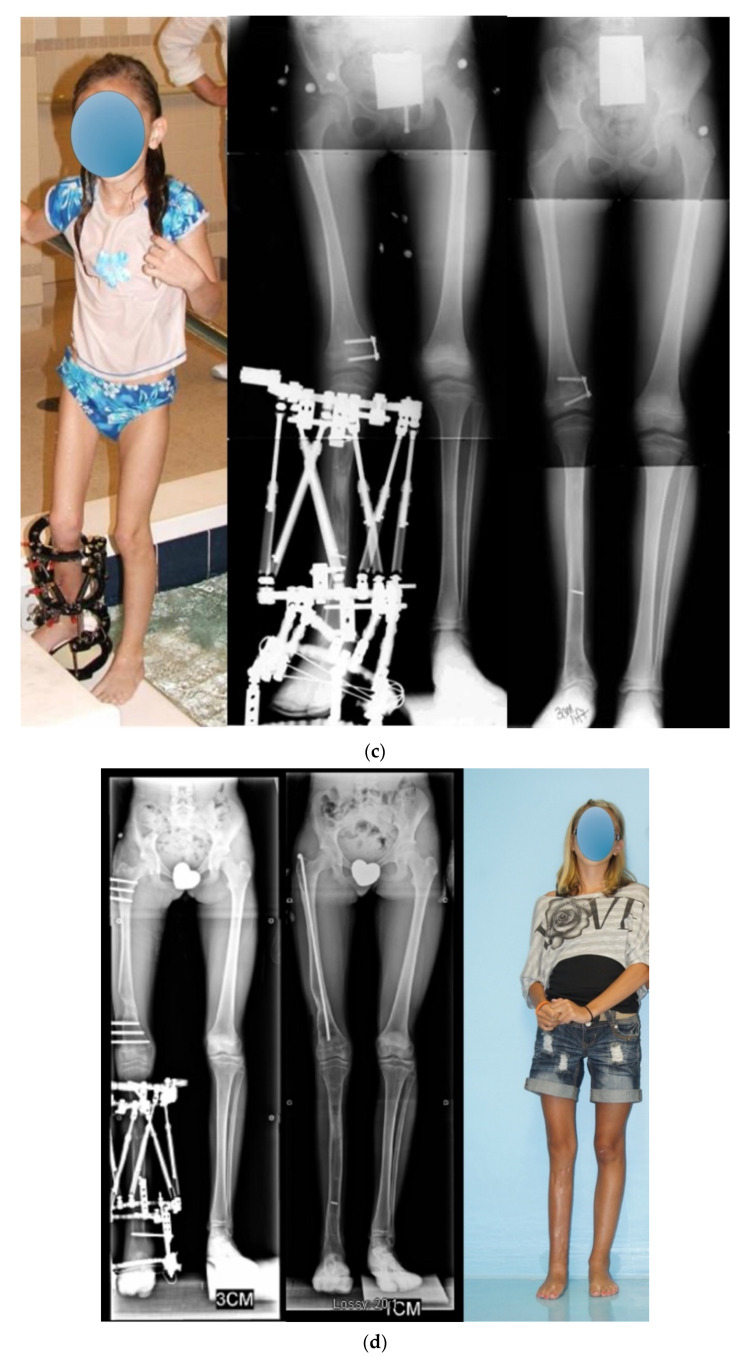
(a) Preoperative radiographs and photographs of an 18-month-old girl born with Paley type 3a FH. She has fixed equinovalgus of the foot and a procurvatum-valgus diaphyseal tibial deformity. (b) Photograph (left) and radiograph (middle left) after the SUPERankle procedure combined with application of a circular external fixator for 5 cm of lengthening. Radiographs of the lower limbs after removal of the external fixator (right middle and right). (c) Photograph (left) and radiograph (middle) showing second lengthening of tibia at age 8, using computer dependent external fixator. She is shown doing pool therapy. Radiograph after removal of external fixator with correction of distal femoral valgus malalignment by hemiepiphysiodesis (right). (d) Radiograph showing the third and final lengthening of the tibia with lengthening of the femur both with external fixation at age 13. Radiograph after removal of the external fixators showing excellent alignment and equalization of limb lengths (middle). Final photograph at age 16 years after skeletal maturity with equal leg lengths and excellent function.
